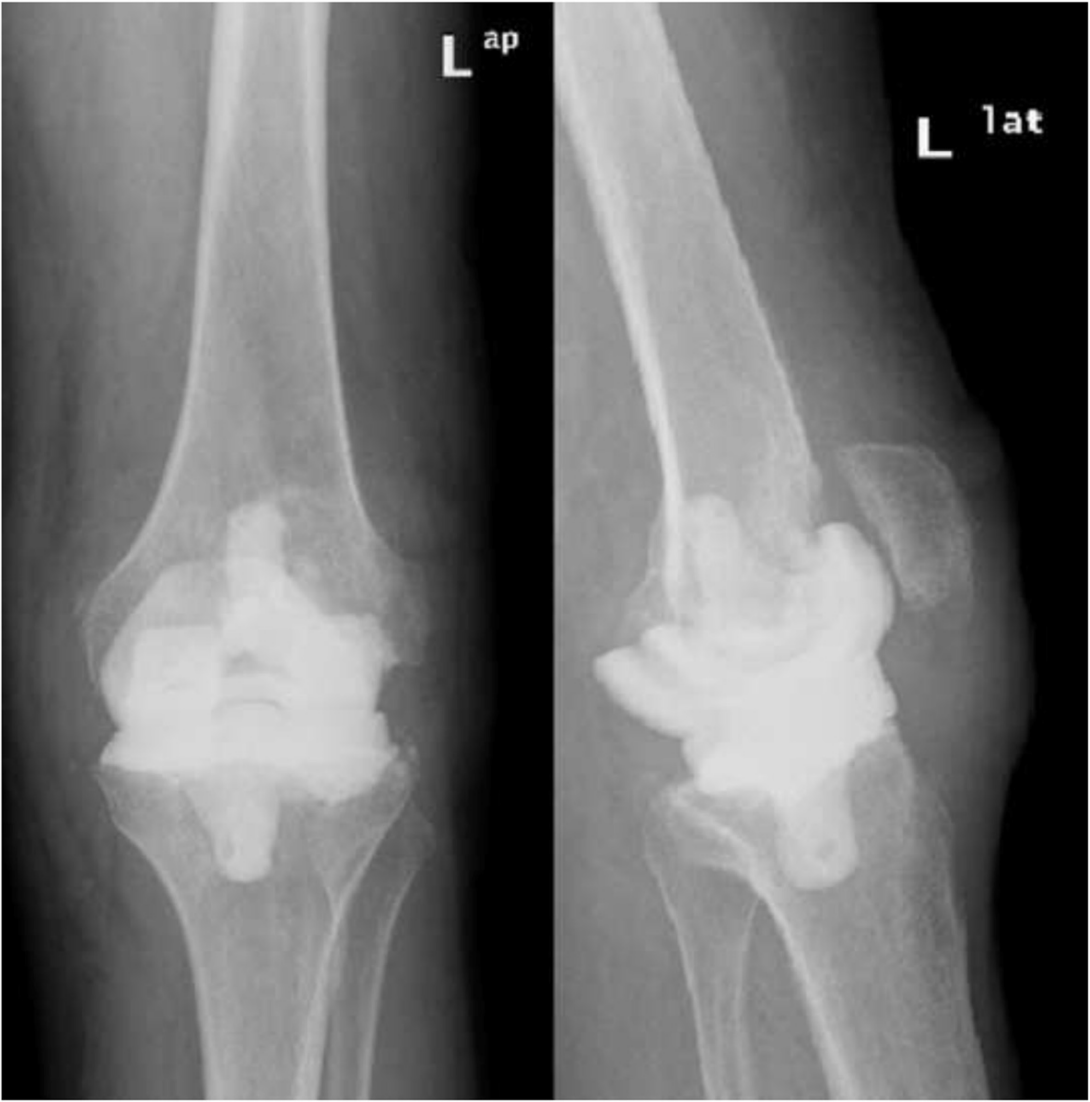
X-rays (AP and lateral view) after 1^st^ stage of surgery treatment with implantation of knee Spacer
